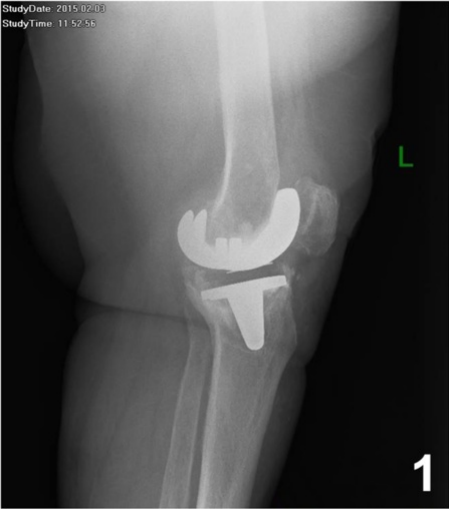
Radiography of the left knee shows a loosening of the prosthesis
